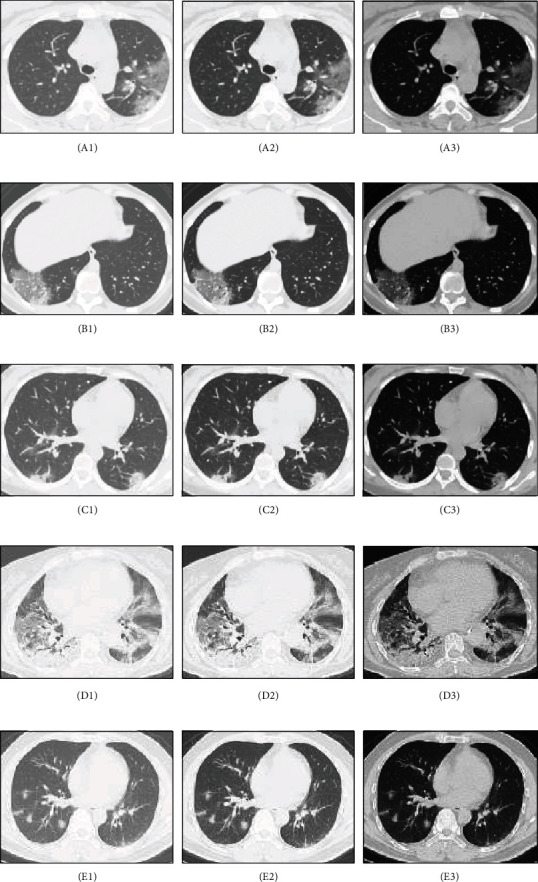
Image enhancement over FEV for chest CT images of COVID-19 pneumonia patients.
